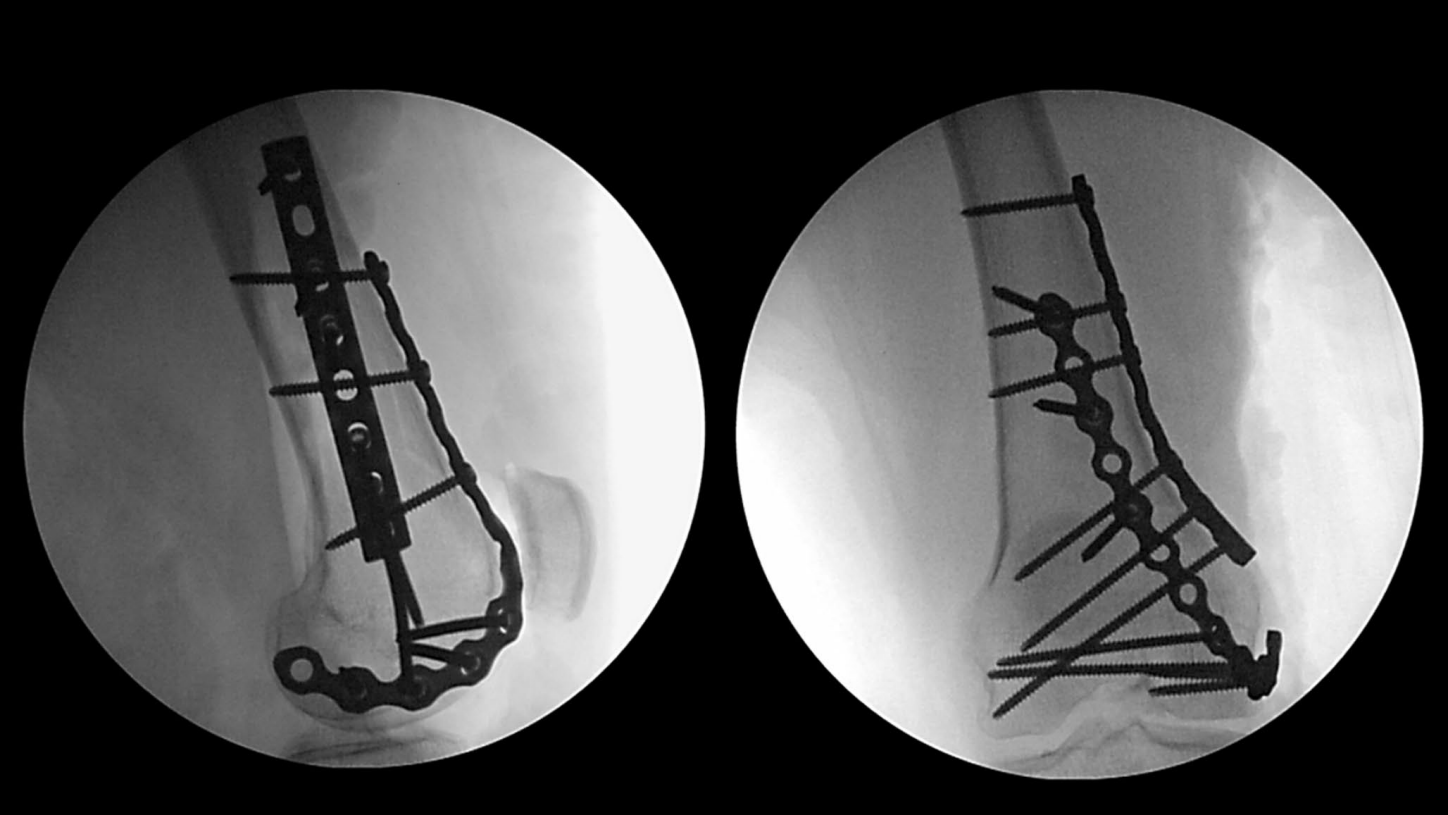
Final intraoperative anteroposterior and lateral radiographs displaying medial femoral condyle with near anatomic reduction
